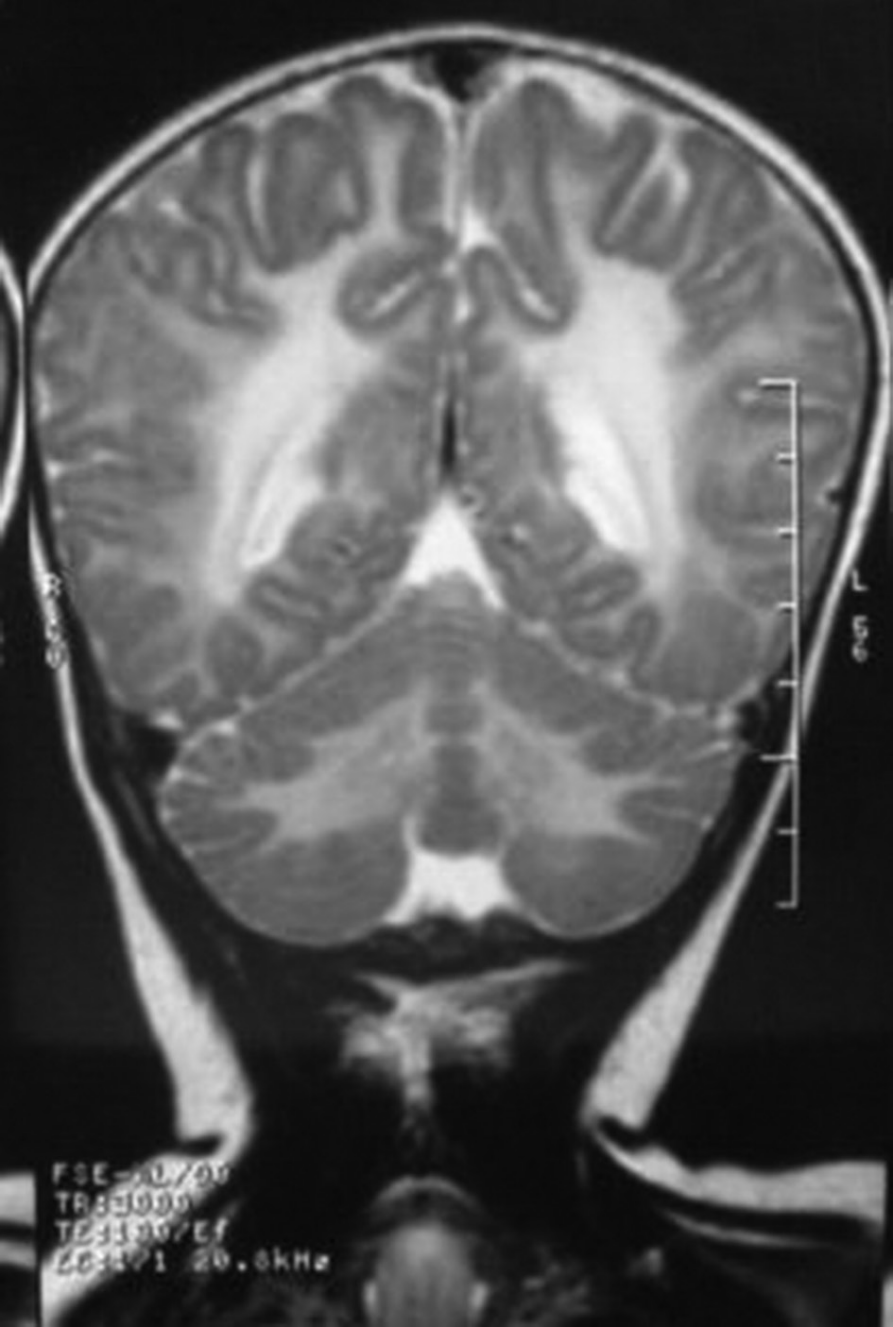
Coronal T2 weighted MRI shows diffuse increased signal intensity in the white matter, in the supra- and infratentorial regions. Symmetric increased signal intensity is seen in both hemispheres.
